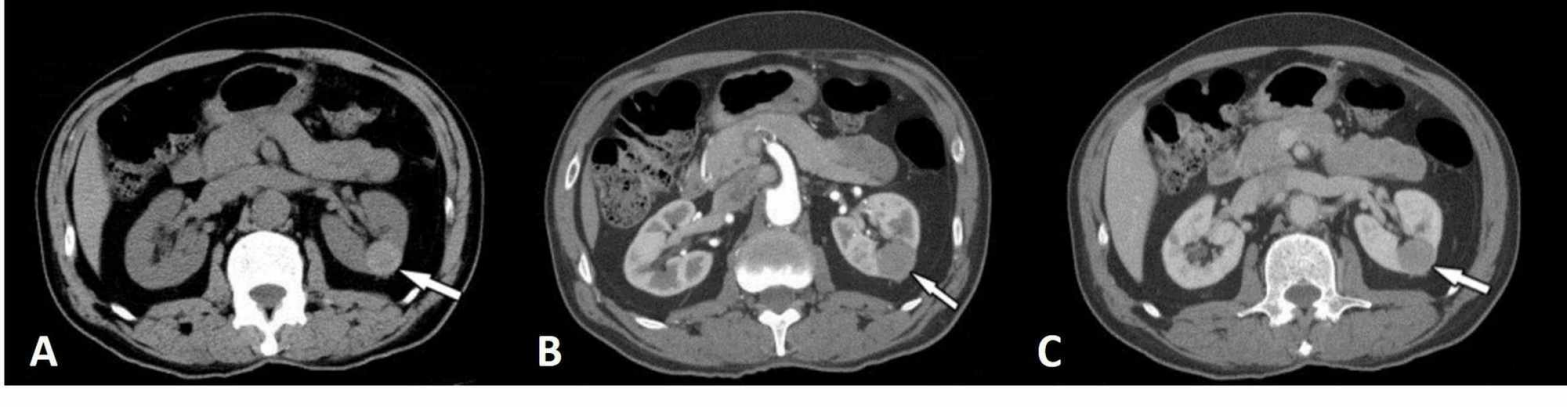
Papillary renal cell carcinoma (arrows) Unenhanced (a), corticomedullary-phase (b), and nephrographic-phase (c) computed tomography images show a precontrast homogeneous hyperattenuating, hypovascular mass lesion without significant enhancement in the left kidney.
